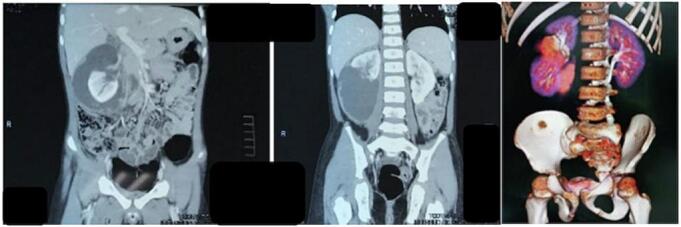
Abdominal CT scan of the patient.
